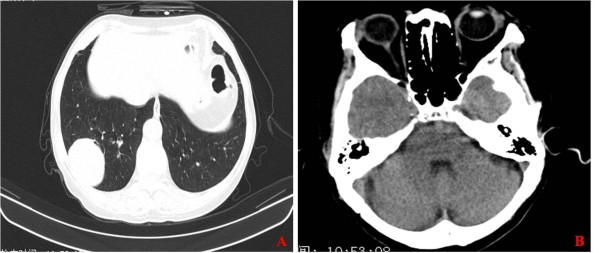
Chest and cranial CT of the second patient: A chest Computed tomography (CT) revealed a spherical mass in the right lung lower lobe (Figure 3A), and cranial CT showed normal (Figure 3B).
